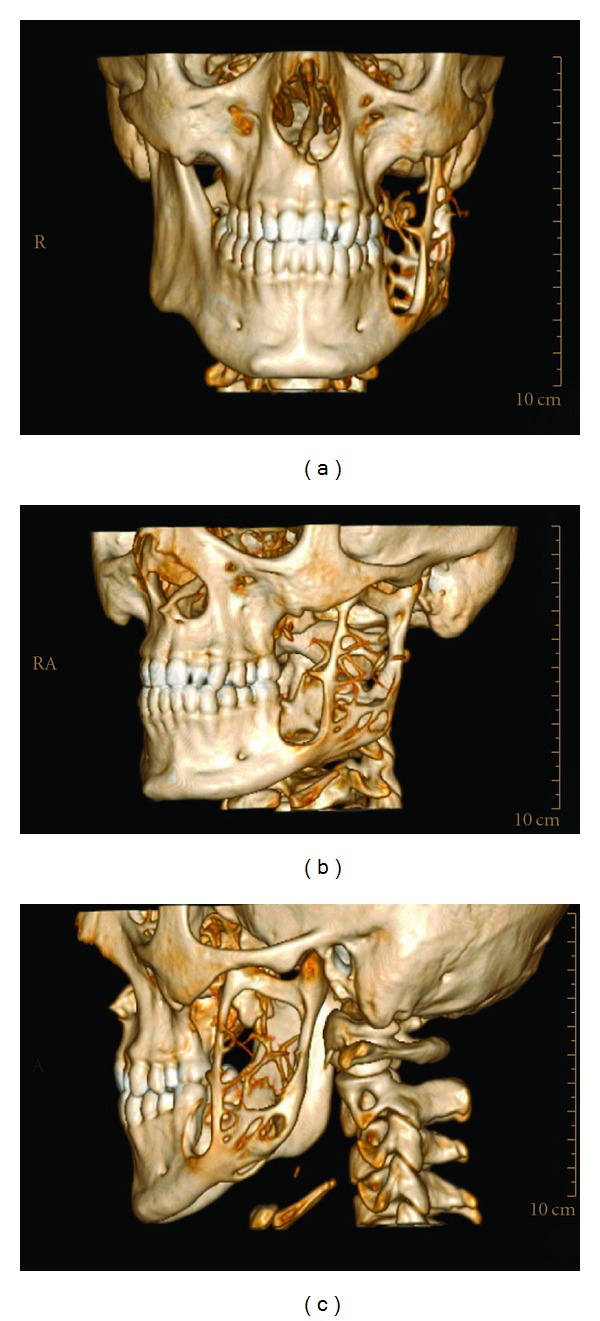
3D CT frontal (a) sagittal (b,c) images demonstrate the lingual, buccal cortical and basis of mandibular erosion with a multilocular bony defect like soup bubble appearance.
